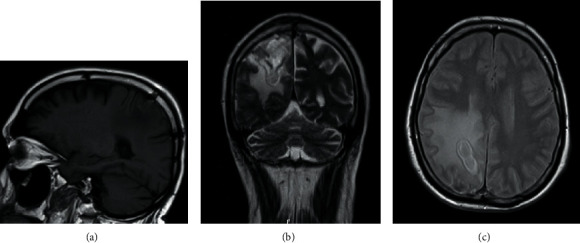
CE-MRI at 12 hospitalisation day.
